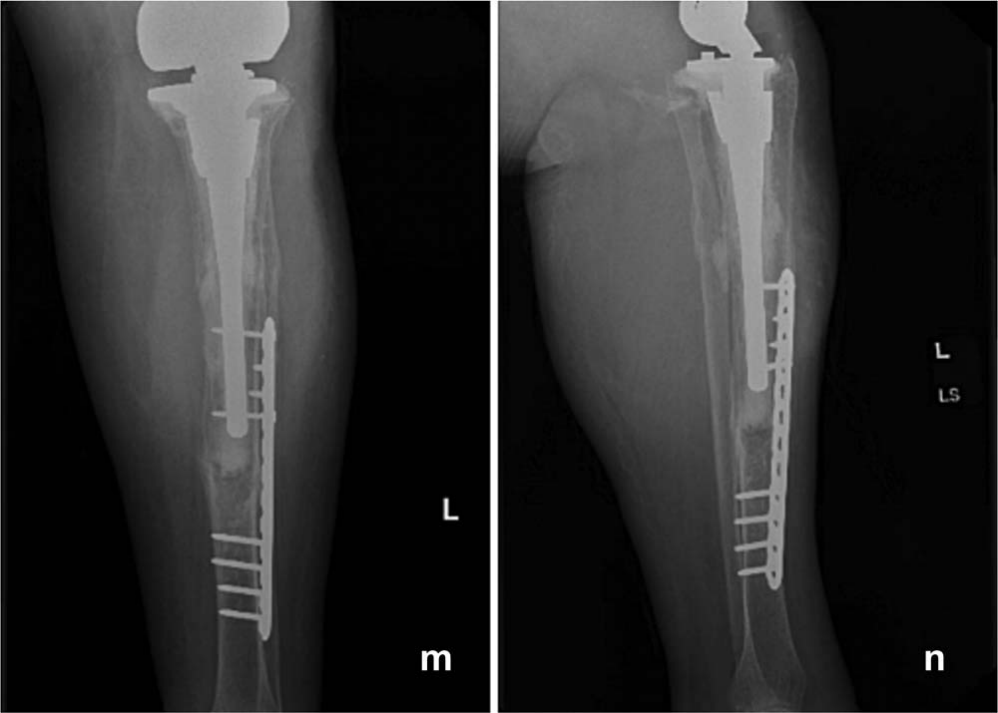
Follow-up anteroposterior (m) and lateral (n) radiographs at 1 year demonstrating intact tibial fixation following fracture repair and a stable total femur construct without evidence of loosening or reinfection.
